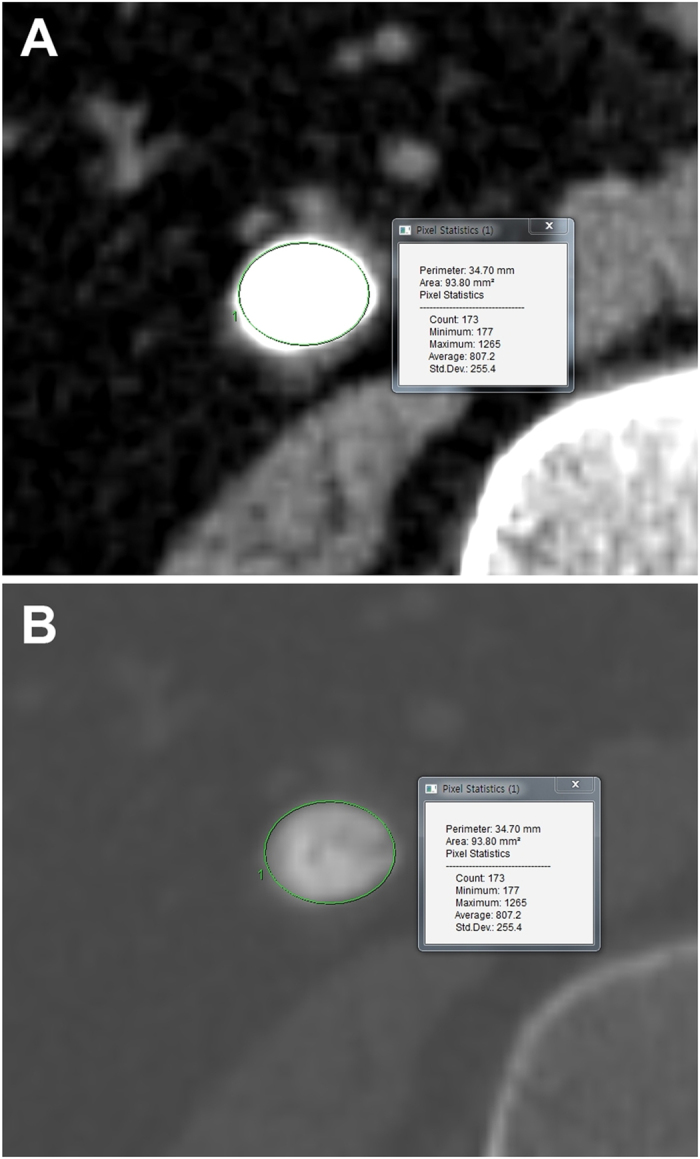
Measurement of stone heterogeneity index (SHI) on axial images of non-contrast computed tomography (NCCT). (A) Soft tissue setting view, (B) Bone setting view (Mean stone density = 807.2 HU and Stone heterogeneity index = 255.4 HU).
